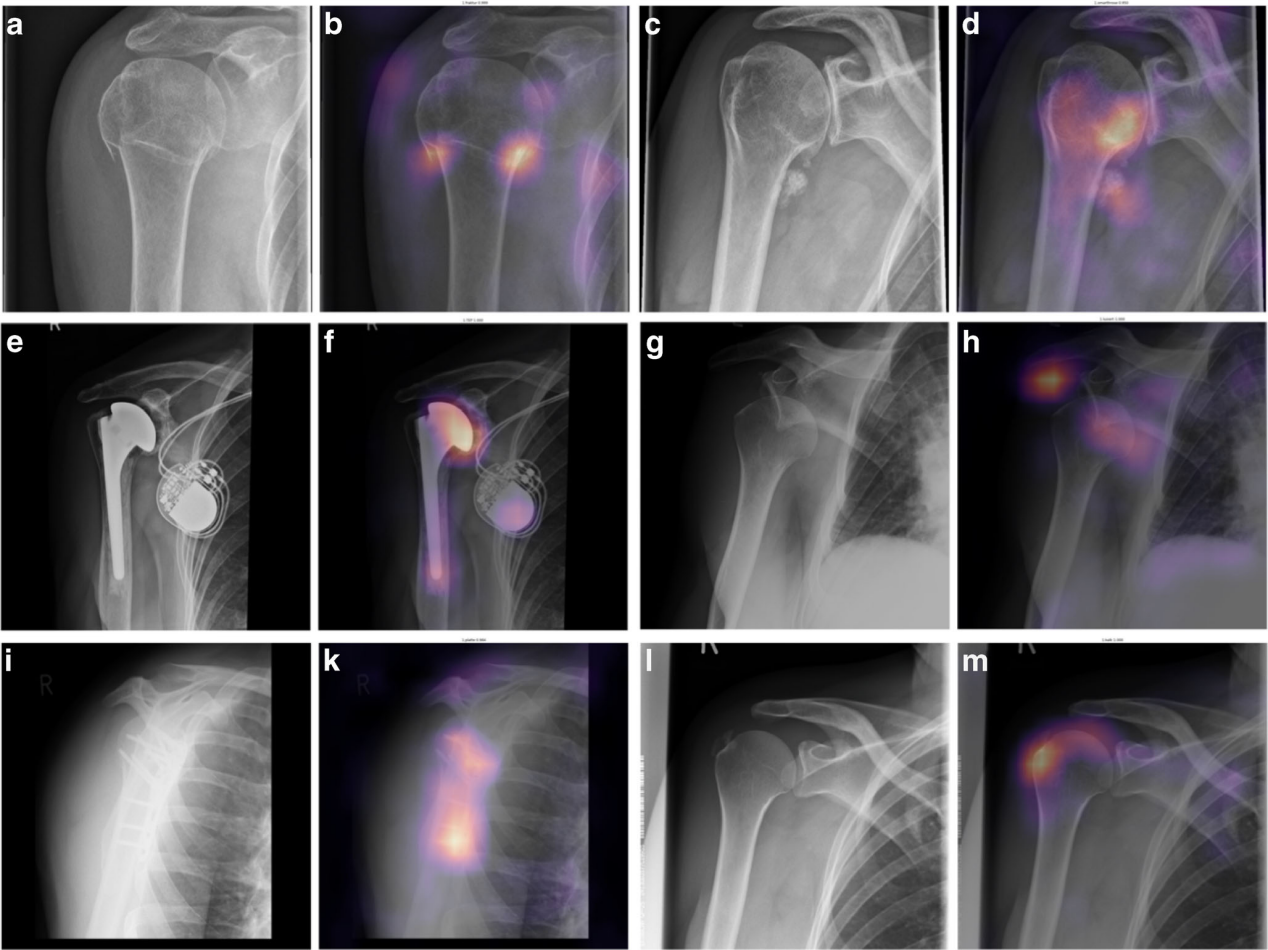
Examples of correct predictions highlighted by Gradient-weighted Class Activation Mappings (Grad-CAMs) for radiographs with only one finding. Images A, C, E, G, I, and L are the original images showing a fracture (A), osteoarthritis (C), an endoprosthesis (E), dislocation (G), osteosynthesis material (I), and calcification (L). Images B, D, F, H, K, and M are the corresponding Grad-CAMs. Grad-CAMs highlight image areas that were important to the model’s decision. They can be used to verify that a pathology was correctly identified and that the decision was not based on image noise (e.g., due to overfitting) or a different finding (e.g., a model that should detect a fracture but instead detects only osteosynthesis)
